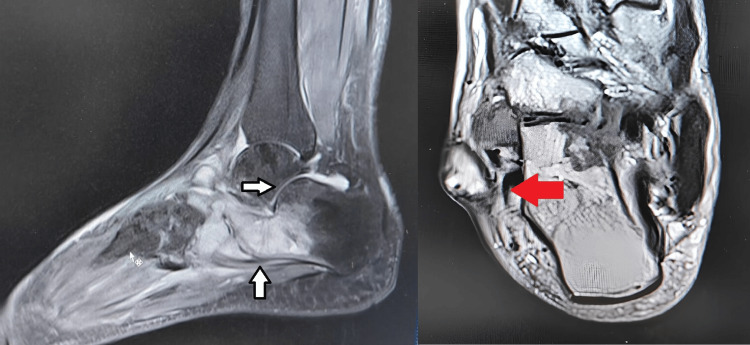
MRI of the right foot and ankle (sagittal cut and axial cut). Bone marrow signal changes were noted over the anterior calcaneum and cuboid, with fluid collection in the ankle joint. The red arrow shows a loculated abscess with granulation pointed out over the lateral aspect measuring 2 x 3 cm.
